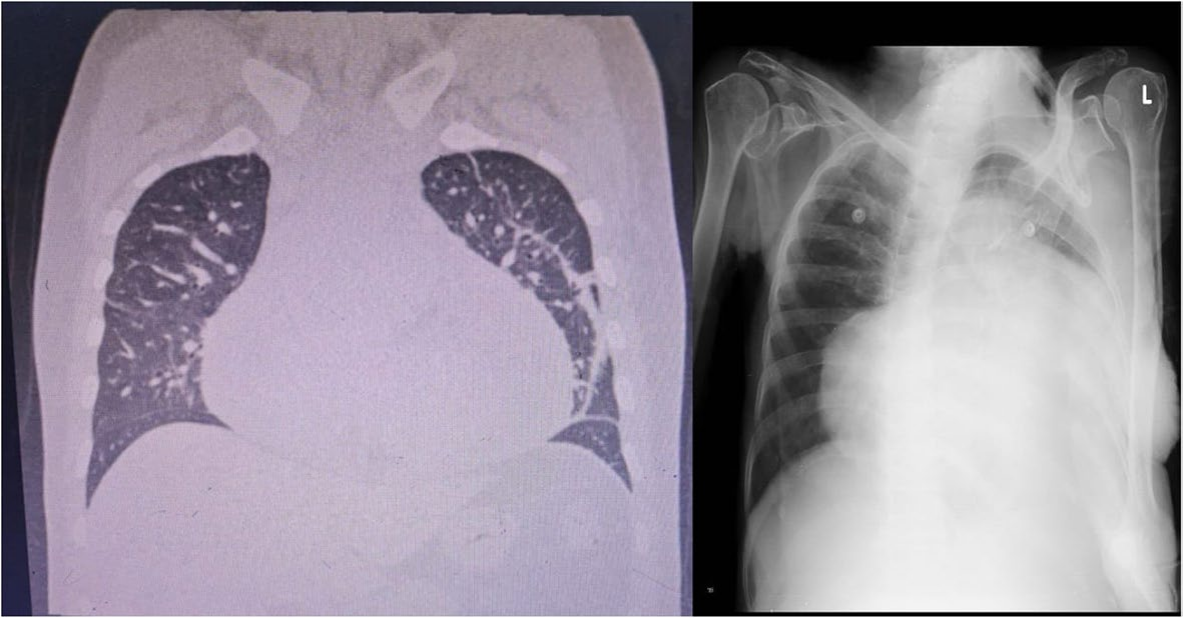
HRCT & Chest X-Ray showing gross cardiomegaly
